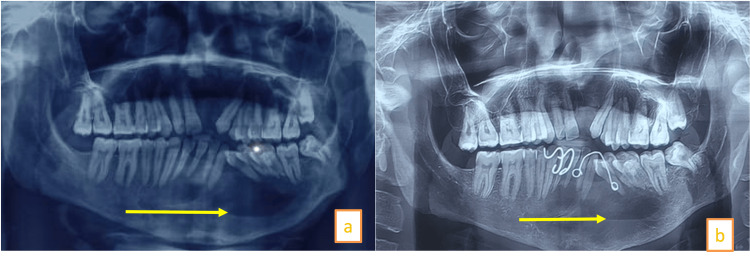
(a) OPG at six-month follow-up showing a reduction in the size of the cystic cavity. (b) 1.5-year follow-up OPG showing a significantly reduced cystic cavity OPG: Orthopantomogram
